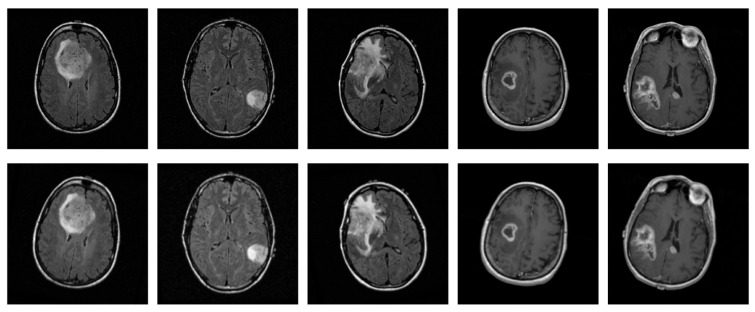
Representative original MRI slices from the training set (top row) and their corresponding augmented versions (bottom row), generated using random rotation, flipping, and shifting.
